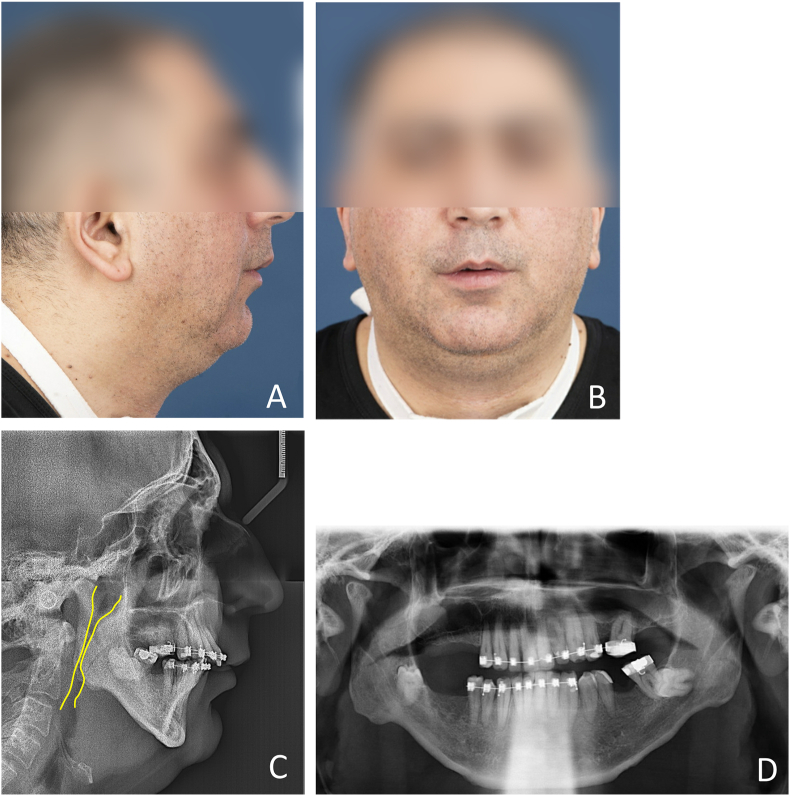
Before maxillomandibular advancement surgery. A, profile view of the extra-oral photograph; B, frontal view of the extra-oral photograph; C, lateral cephalogram; D, panoramic radiograph.
